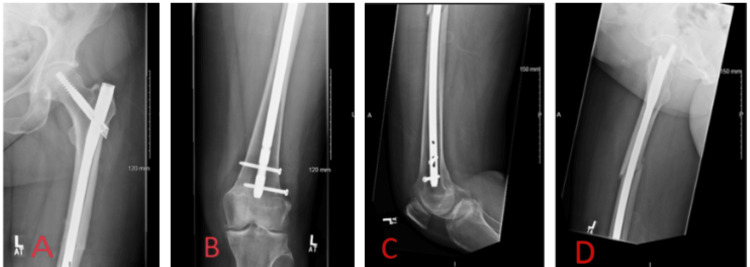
Postoperative X-rays after the first surgery from the hospital providing primary care A: Anteroposterior X-ray of the left proximal femur; B: Anteroposterior X-ray of the left distal femur; C: Lateral X-ray of the left distal femur; D: Axial X-ray of the left proximal femur
